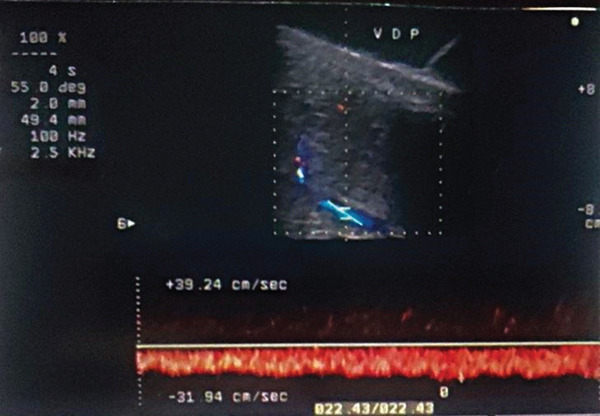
Normal cavernosal artery inflow on dynamic penile color Doppler ultrasonography.
